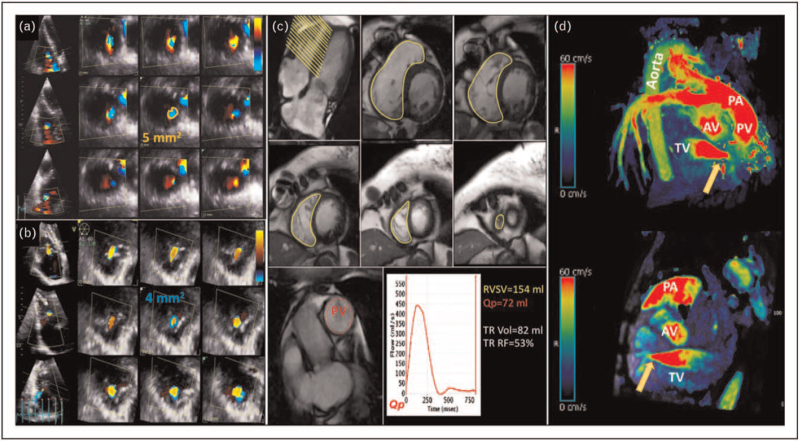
Measurement of three-dimensional vena contracta by transthoracic echocardiography in a patient with functional tricuspid regurgitation (a). Measurement of three-dimensional effective regurgitant orifice area by transthoracic echocardiography in a patient with severe tricuspid regurgitation (b). Quantification of tricuspid regurgitant volume and fraction using cardiac magnetic resonance (c). Regurgitant volume is derived by the difference between the right ventricular stroke volume (yellow) and the forward pulmonary valve stroke volume (red). Assessment of tricuspid regurgitation (orange arrows) using cardiac magnetic resonance 4-D flow images with flow velocity encoded in all directions (d; courtesy of Dr Giuseppe Muscogiuri, Centro Cardiologico Monzino IRCCS, Milan, Italy). PA, pulmonary artery; PV, pulmonary valve; TV, tricuspid valve.
